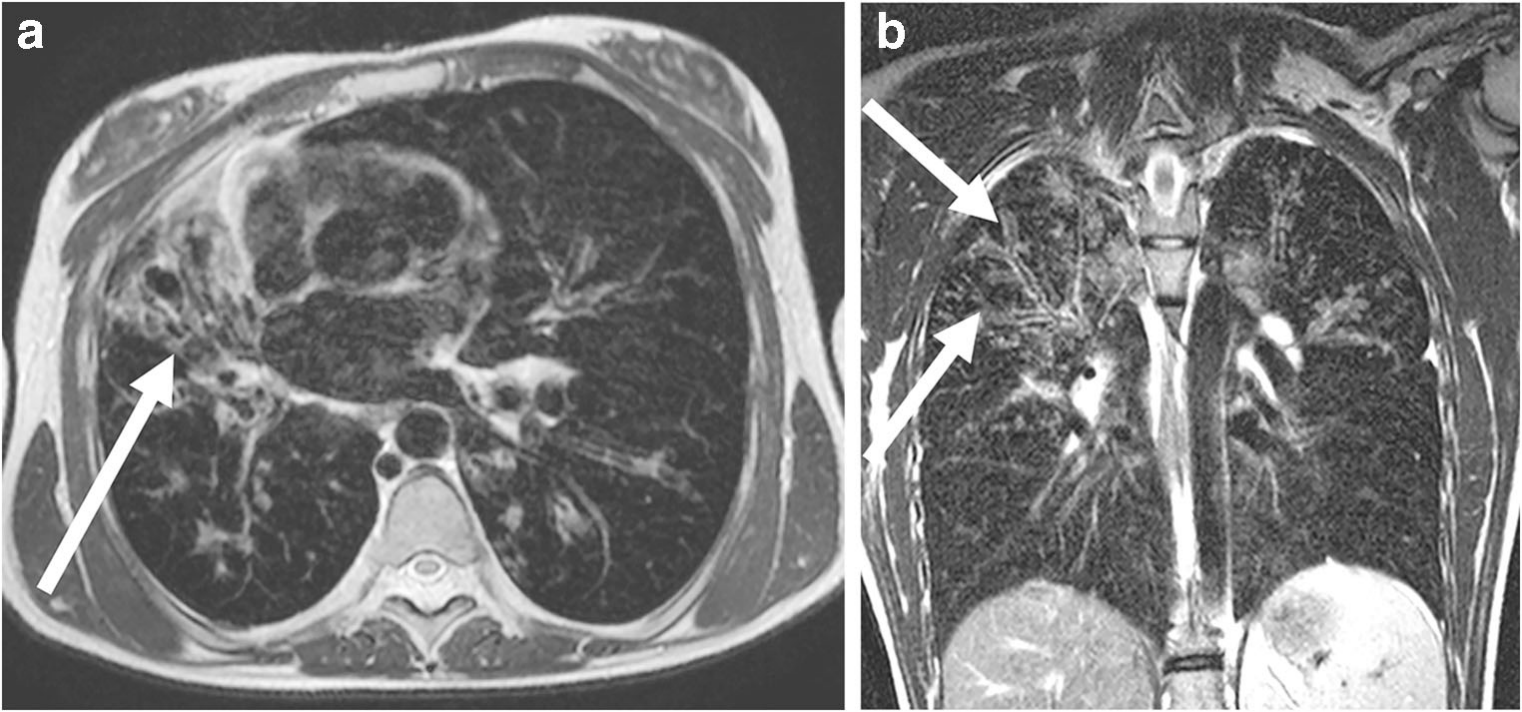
Lung MR in a 14-year-old girl with cystic fibrosis. a, b T2-weighted turbo spin-echo axial (a) and coronal (b) images show multiple bronchiectases among scarred strands, infiltrations and mucus plugging in the upper and middle lobes (*arrow* in a). Pulmonary scarring leads to thoracic asymmetry. Smaller pneumonic infiltrates (*arrows* in b) can be seen. Various scoring systems have been published and correlate well with the modified Bhalla CT score and the Chrispin–Norman score
